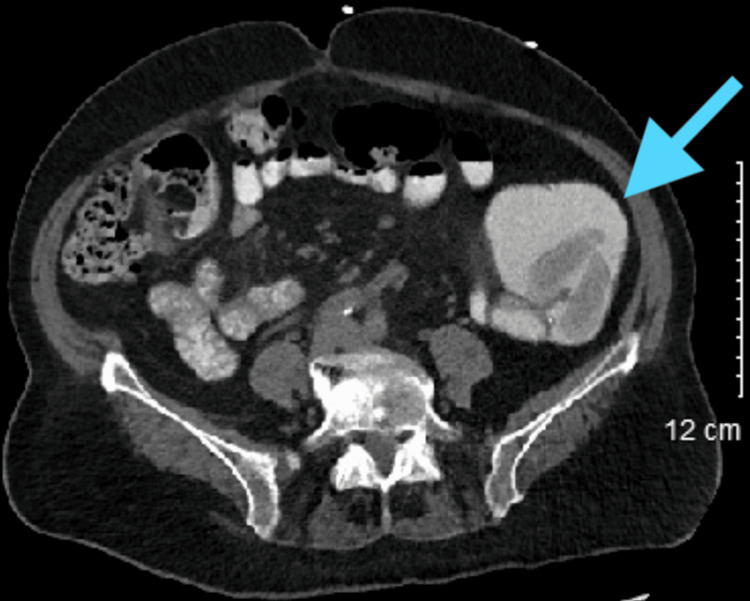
An abdominal CT scan with oral contrast demonstrating the enteroliths found intraoperatively.
